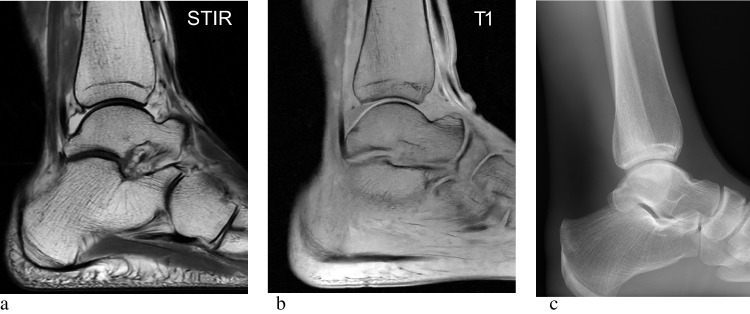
SABM in a 32-year-old female with a low BMI secondary to anorexia nervosa. T1-weighted images show mildly hypointense signal of the subcutaneous tissues (a). Short inversion time inversion recovery (STIR) sequence of the ankle (b) demonstrates reduced thickness and abnormal hyperintensity of the subcutaneous adipose tissues. The lack of adipose tissue and loss of fat planes is also demonstrated on the corresponding plain radiograph (c)
